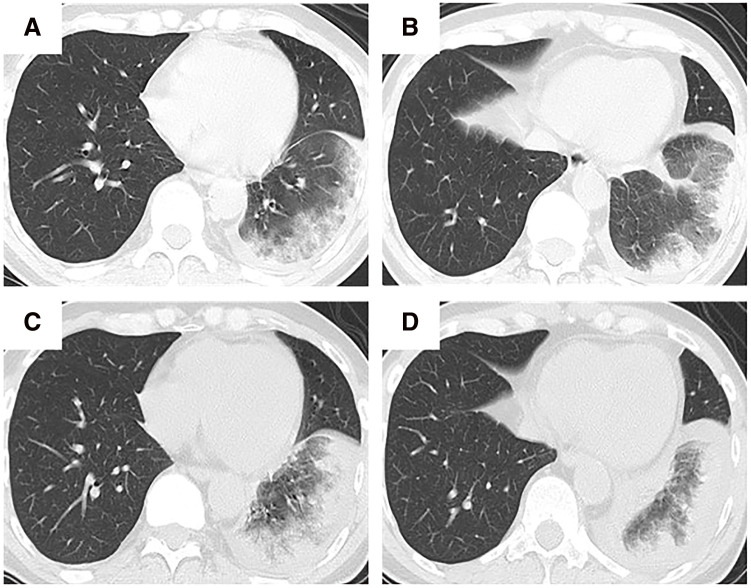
Clinical course of lung infiltration after catheter ablation. (A and B) Chest computed tomography (CT) scans 6 months after catheter ablation just before the beginning of antibiotic therapy. (C and D) Chest CT scans 10 days after antibiotic therapy.
